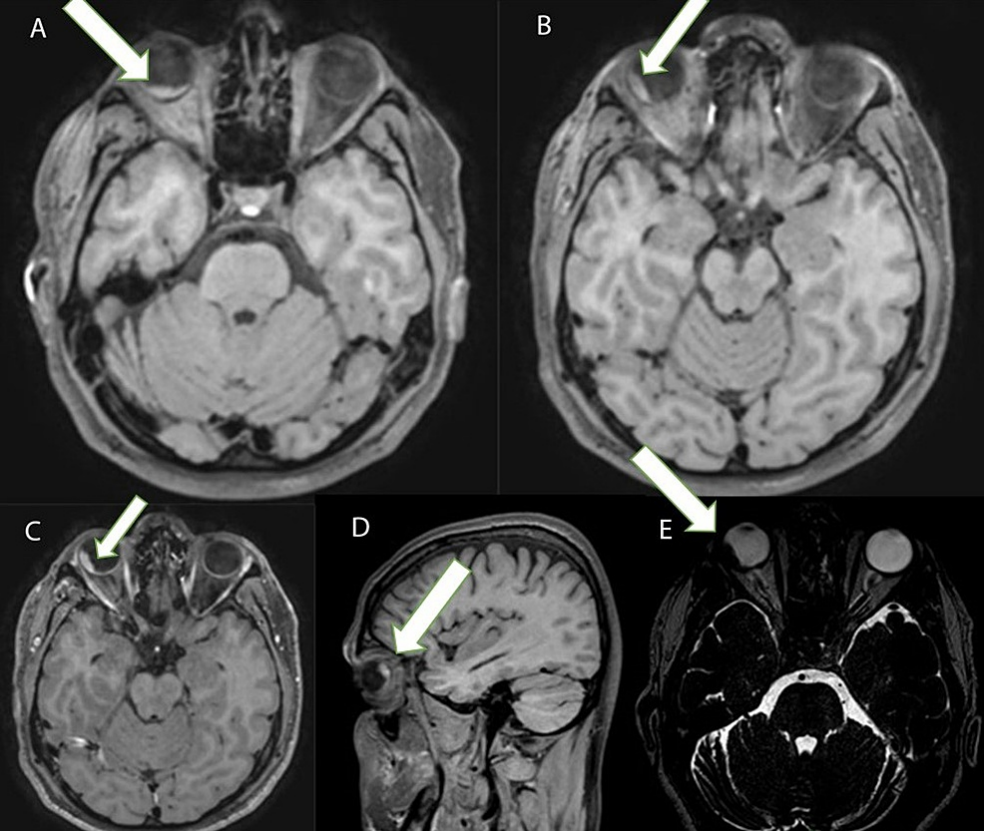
Head MRI showing T1 and T2 weighted images. A: T1 weighted image post gadolinium enhancement, axial plane: evidence of retinal detachment in the right eye globe centrally in the lower quadrants. B: T1 weighted image post gadolinium enhancement, axial plane: showing the extension of the retinal detachment posteriorly temporal in the upper quadrant with evidence of a nodular-like hyperintense lesion. Enhancement is extended more than the dimensions of the high signal lesion on T1 in the non-enhancing images. C: T1 weighted image without enhancement, axial plane: showing a hyperintense nodular-like lesion measuring 13 mm in its longitudinal axis and 5 mm in thickness. D: T1 weighted image post gadolinium enhancement, sagittal plane: showing the hyperintense nodular lesion in the temporal upper quadrant. E: T2 weighted image, axial plane: showing a nodular-like lesion of low signal. The arrow indicates melanoma location.
